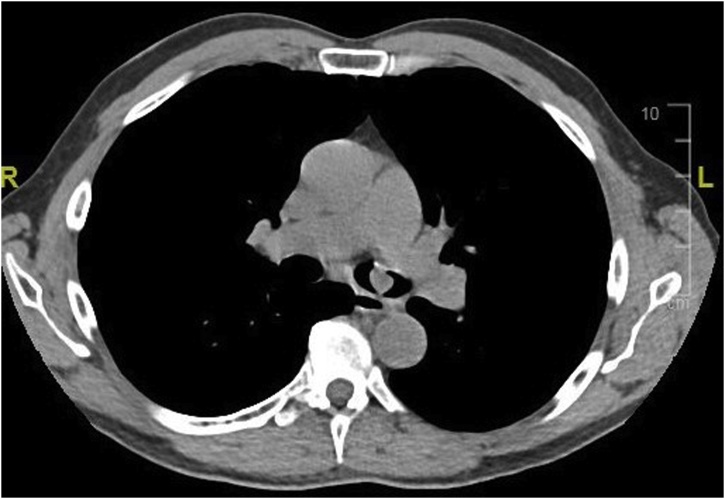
CT chest shows a left endobronchial tumor.
